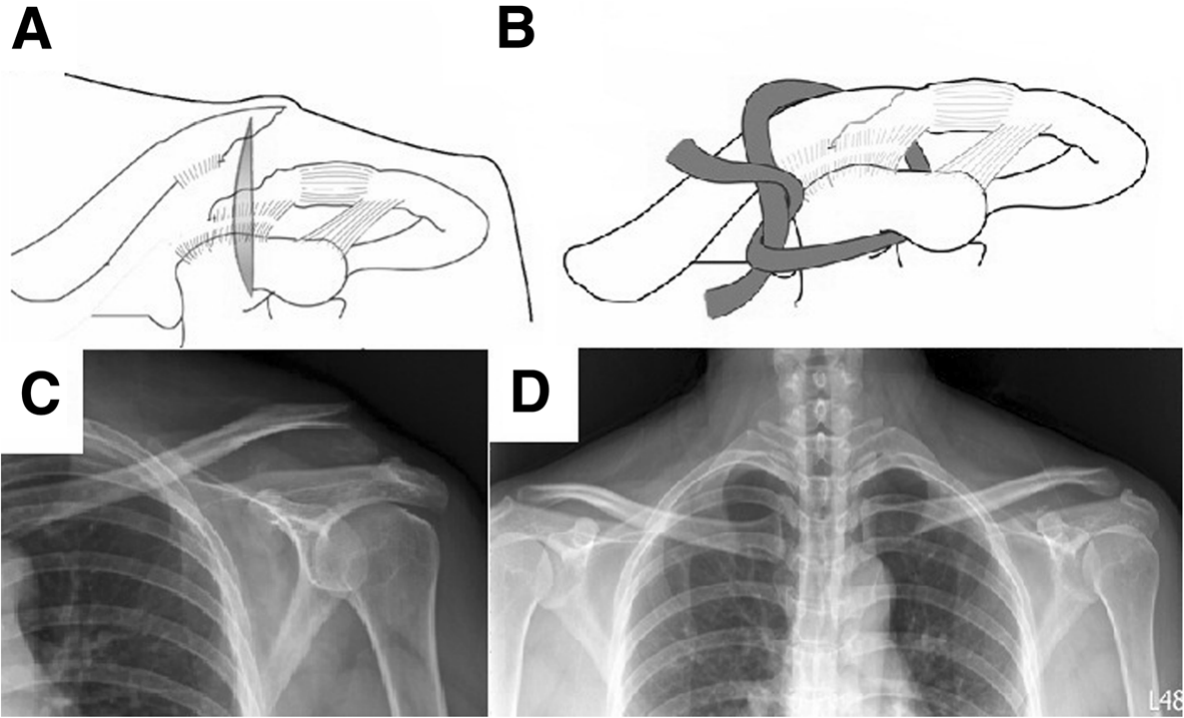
Stabilization by single CC suture fixation with Mersilene tape. Surgical procedure: (A) A longitudinal incision was made above the coracoid process. (B) Mersilene tape was passed around the base of the coracoid process and the medial clavicular fragment and was tied to maintain reduction. Radiography of the injured clavicle: (C) Preoperation. (D) Postfixation.
